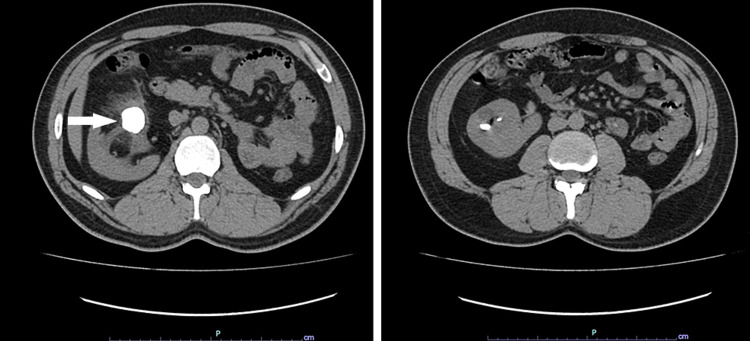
Computed tomography abdominal scan of a 31-year-old male showing right crossed fused renal ectopia with a large 2.7-cm calculus with fat stranding and moderate hydronephrosis.
